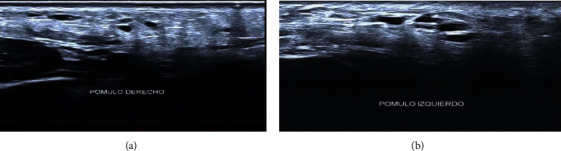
Ultrasound performed with a 24 MHz high-frequency linear transducer, high-resolution Doppler; (a, b), respectively.
